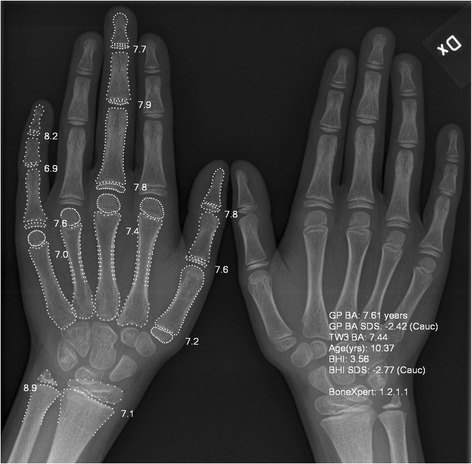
Digital X-ray radiogrammetry of the left and right hand of a patient with JIA. BoneXpert® software was used to perform automated determination of bone age based on the radius, ulna and the bones in ray 1, 3 and 5 (marked with *white dots*). Bone health index (BHI) is based on the measurements of cortical thickness in the three middle metacarpals
